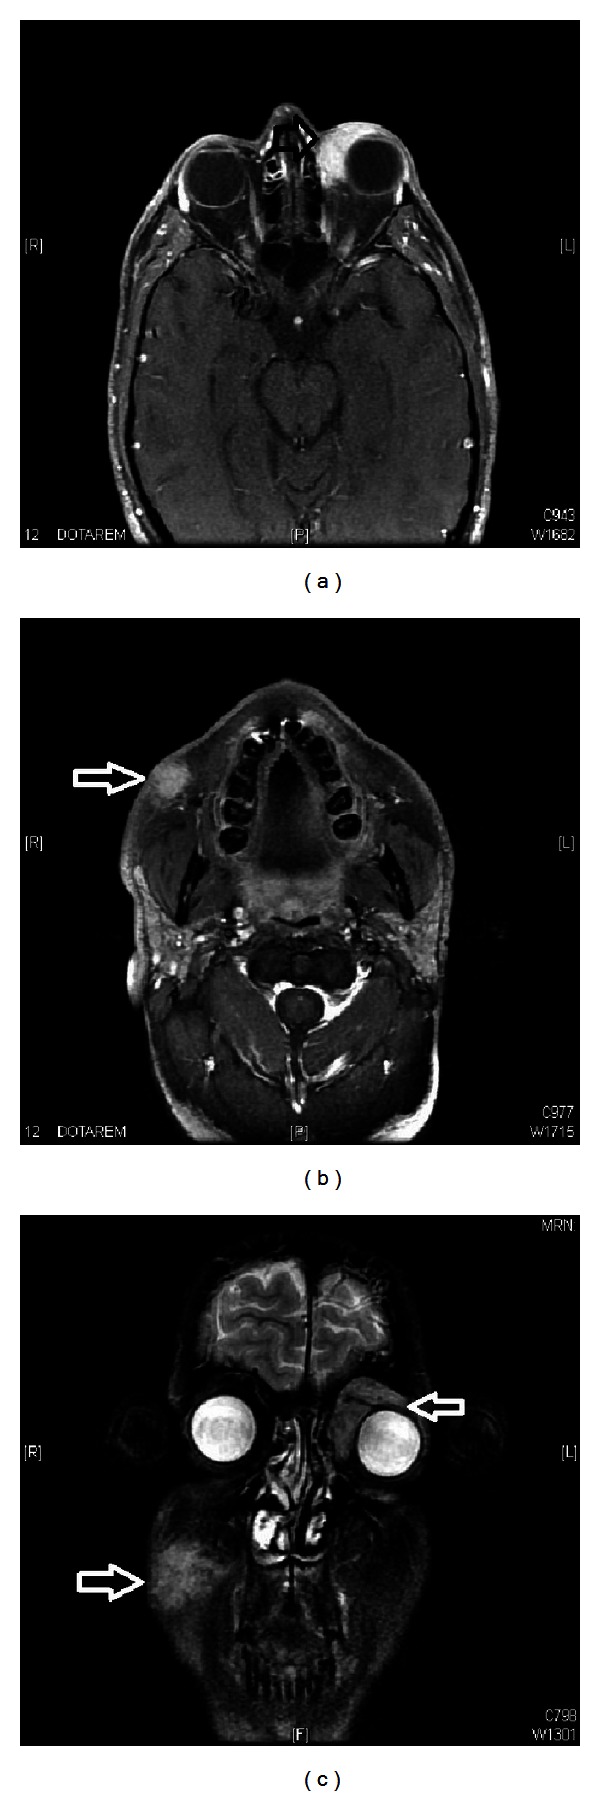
Magnetic resonance imaging (MRI) ((a) and (b)) axial and (c) coronal images of head showing recurrent mass tissue lesion involving the left eyelid, mainly superiorly and medially progressing deeply to surround the superior and medial recti muscles as well as superior oblique muscle mainly at their insertion on the ocular globe and another mass tissue lesion involving the right cheek included within the subcutaneous tissue showing intense enhancement measuring about 3 × 2 cm without obvious deep extension.
